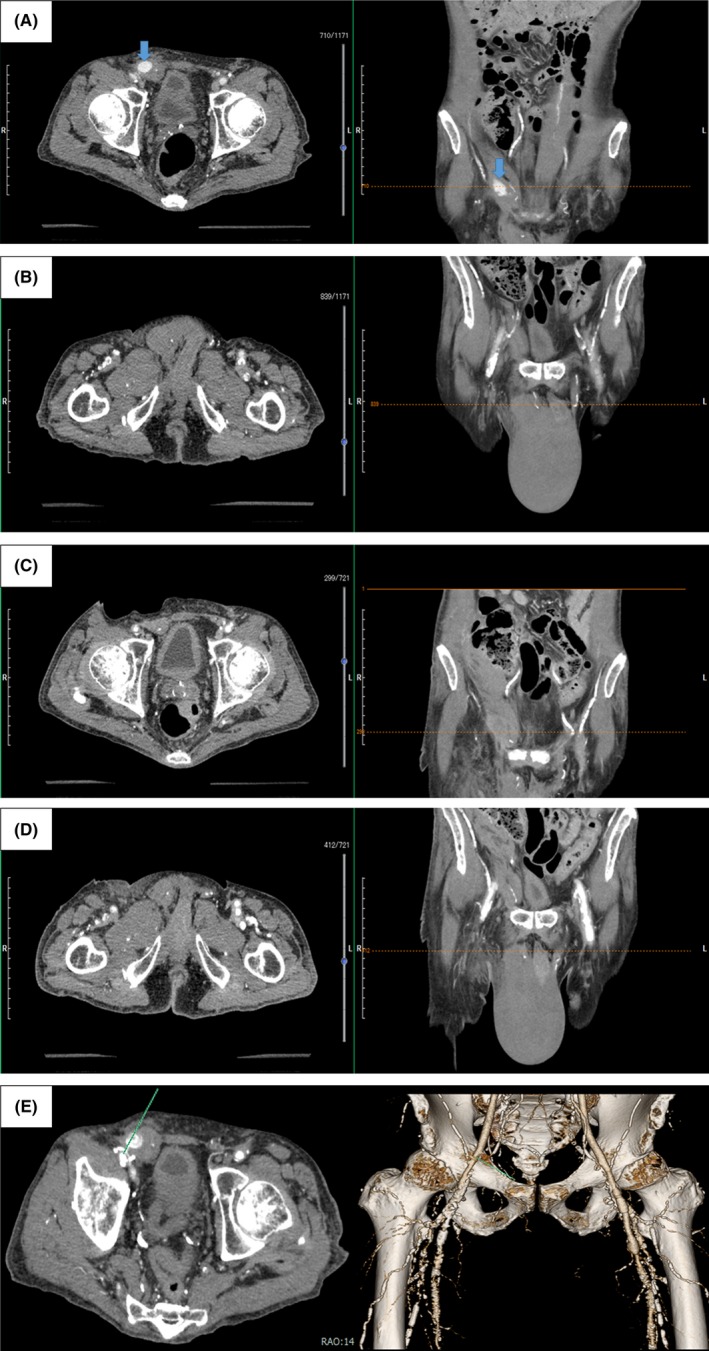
Panel A, Contrast‐enhanced CT after the removal of the femoral sheath and manual compression for 20 min. Blue arrow shows an extravasation of contrast media in the right inguinal canal from the right femoral artery. Panel B, The scrotal hematoma located center of pubis. Panel C, Contrast‐enhanced CT after additional 30 min manual compression. An extravasation of contrast media resolved. Panel D, The scrotal hematoma was not enlarged after additional 30 min manual compression. Panel E, Contrast‐enhanced CT after the removal of the femoral sheath and manual compression for 20 min. Green line is a speculated puncture line. Green line penetrated both the inguinal canal and the femoral artery
